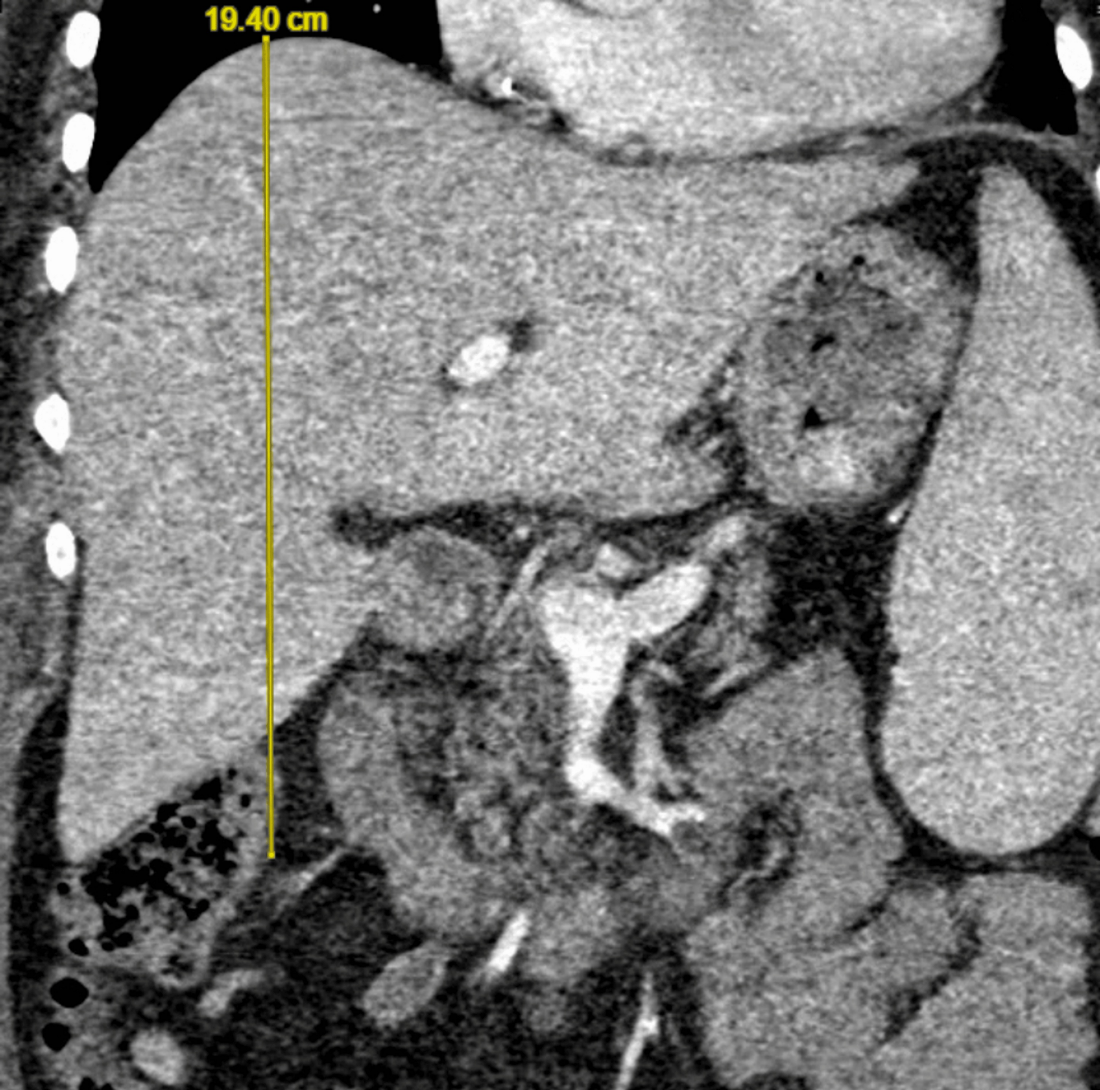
CT scan of the abdomen demonstrating hepatomegaly.
